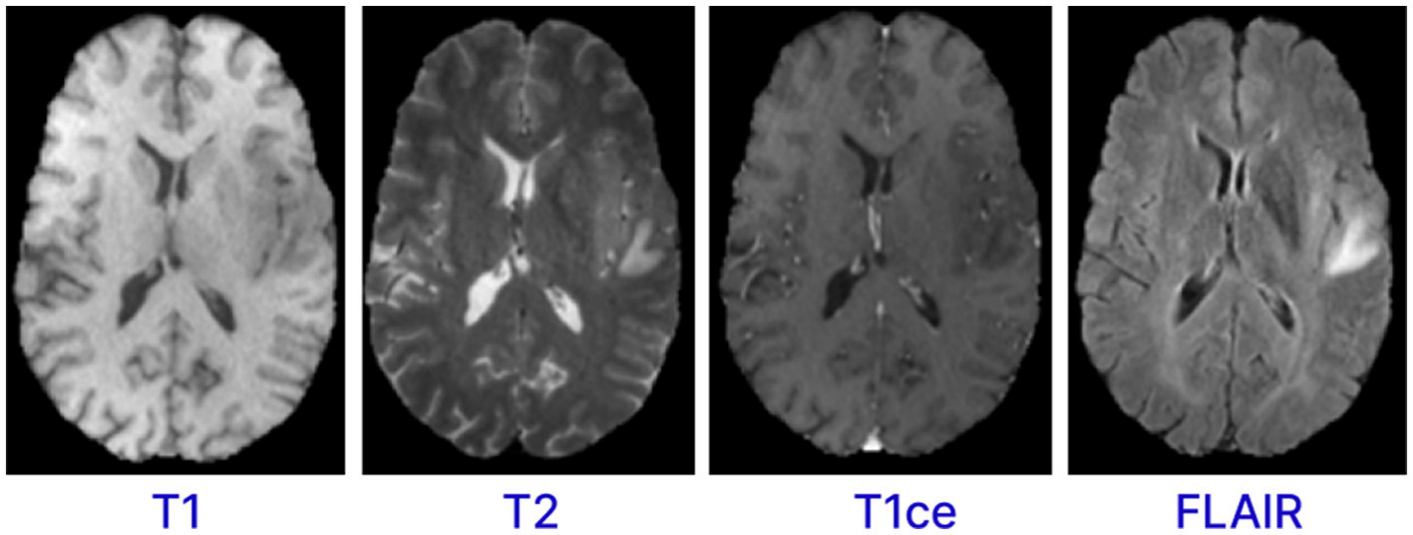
MRI sequences for brain.
